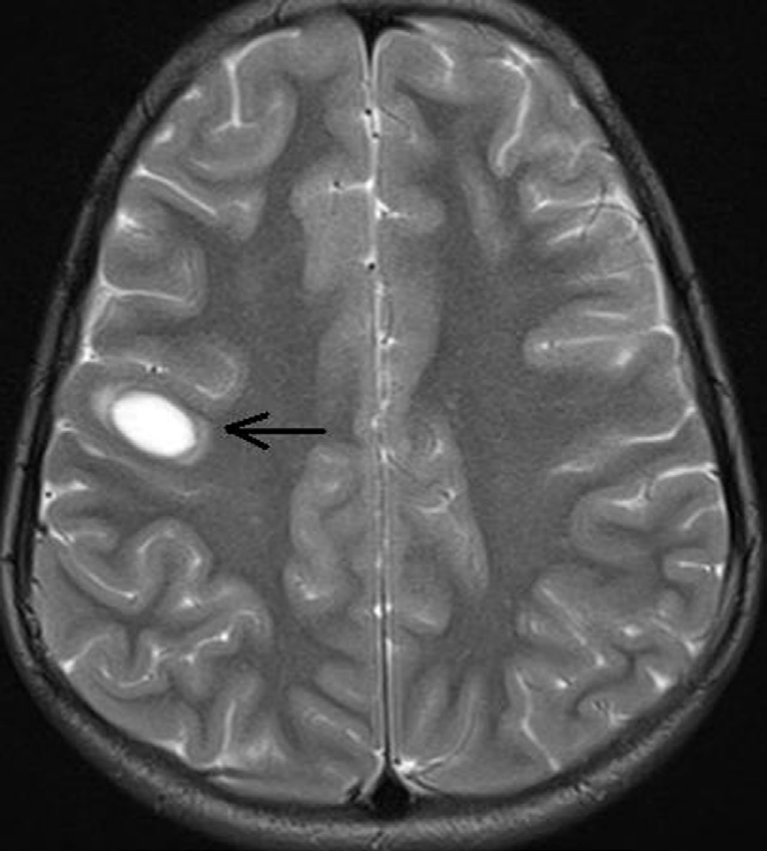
MRI screening of brain showing single focal well-circumscribed hyperintense lesion (arrow) in T2 sequence at right subcortical frontal region (at gray white interface).
